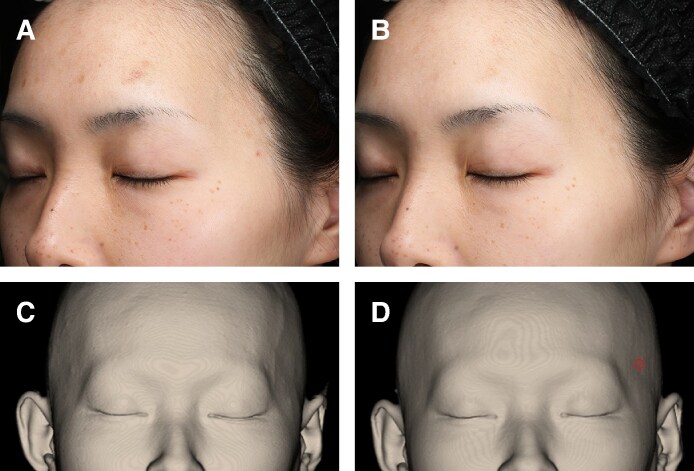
Photographs of the neuromuscular electrical stimulation (NMES)-applied side (A) at baseline and (B) week 8 in a 40-year-old female participant, showing a visible reduction in temporal hollowing after treatment. Hair-removed three-dimensional computed tomography (CT) images of the temporal region (C) at baseline and (D) week 8, respectively. Improved temple contour due to an increase in temporal muscle volume is evident on the NMES-applied side (red circle). Specifically, temporal muscle thickness increased from 5.07 to 5.47 mm (+7.89%), and the depth of temporal hollowing improved from −1.83 to −1.60 mm (−12.73%). No morphological changes were observed on the control side.
